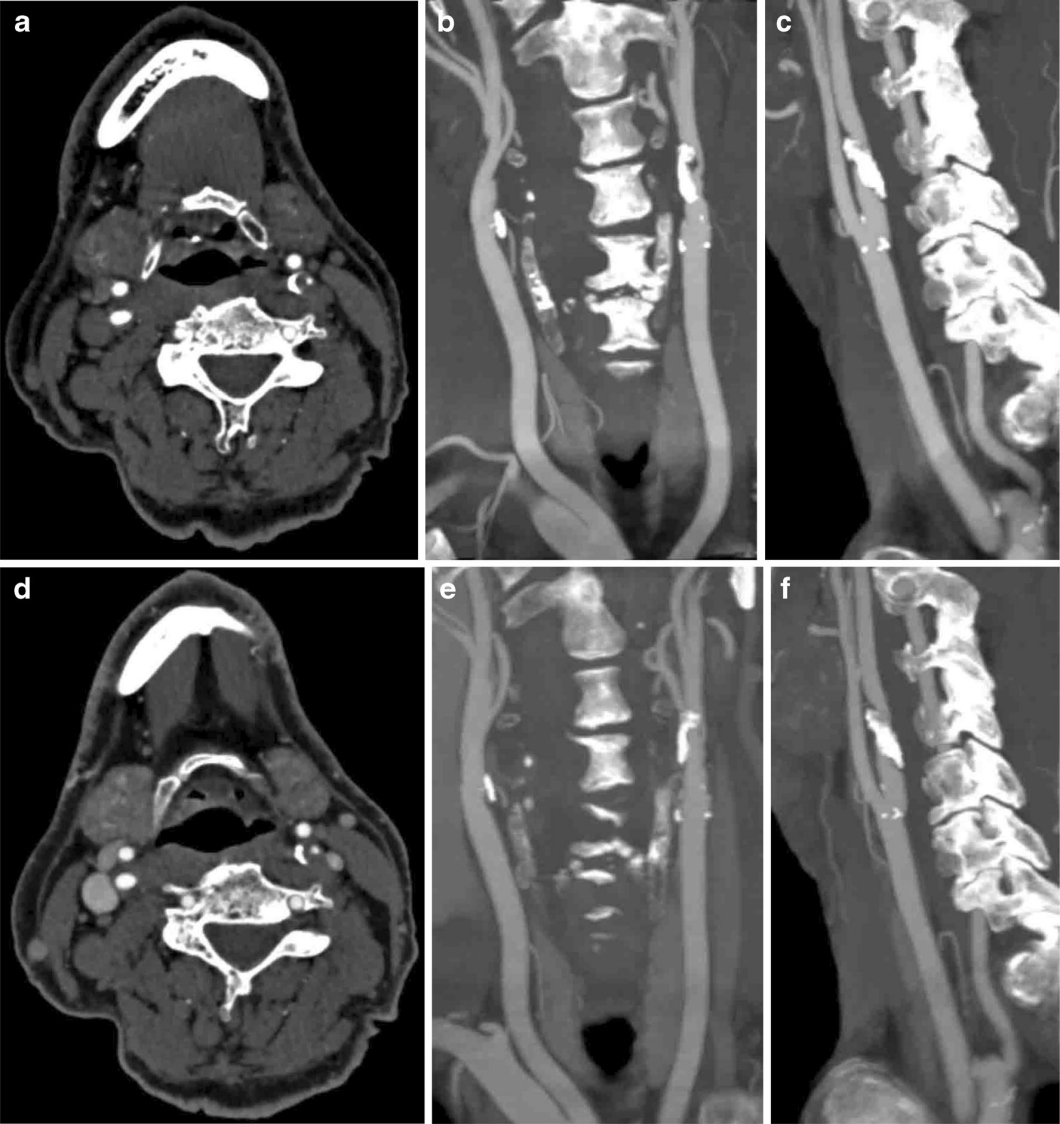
Comparison of the neck CTA of the One-Step Stroke Protocol (a, b and c) and the conventional CTA (d, e and f) in a patient with sudden weakness of the right hand and leg. a and d Axial images at the level above the carotid bifurcation (window centre, 200 HU; window width 700 HU). The left internal carotid artery shows a pinpoint stenoses, plaque and calcifications. In this subject, the origin of the left common carotid artery was missed by 2 cm. Image quality was rated equally good at the level of the carotid bifurcation between the One-Step Stroke Protocol and the conventional CTA protocol. b and e Coronal maximum intensity projections with 10 mm reconstructions; c and f are sagittal maximum intensity projections with 10 mm reconstructions (window centre, 200 HU; window width 700 HU)
